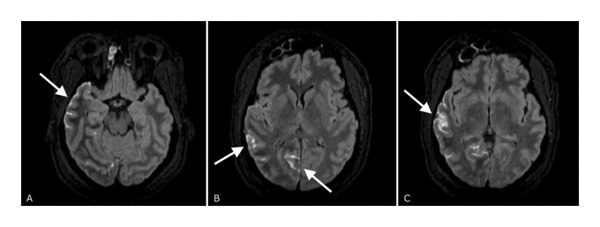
Brain MRI, postcontrast FLAIR sequence, demonstrating gyriform leptomeningeal enhancement over the right temporo‐occipital region (A–C).
